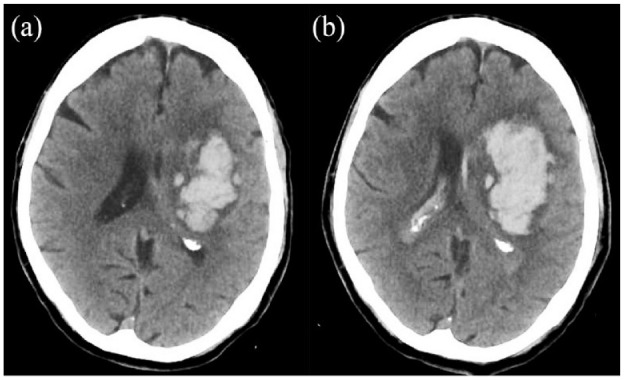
CT brain scans from a patient taking rivaroxaban for atrial fibrillation and a blood pressure on presentation of 183/94. The baseline scan: (a) was 2 h after symptom onset and the second scan and (b) was completed 3 h later. Within 1 h of the baseline scan, systolic blood pressure was lowered to <140 mmHg and 50 IU/kg of four-factor prothrombin complex concentrate was administered. Despite treatment, the haematoma expanded from 52.8 ml (scan a) to 83.1 ml (scan b), an expansion of 30.3 ml (57%).
